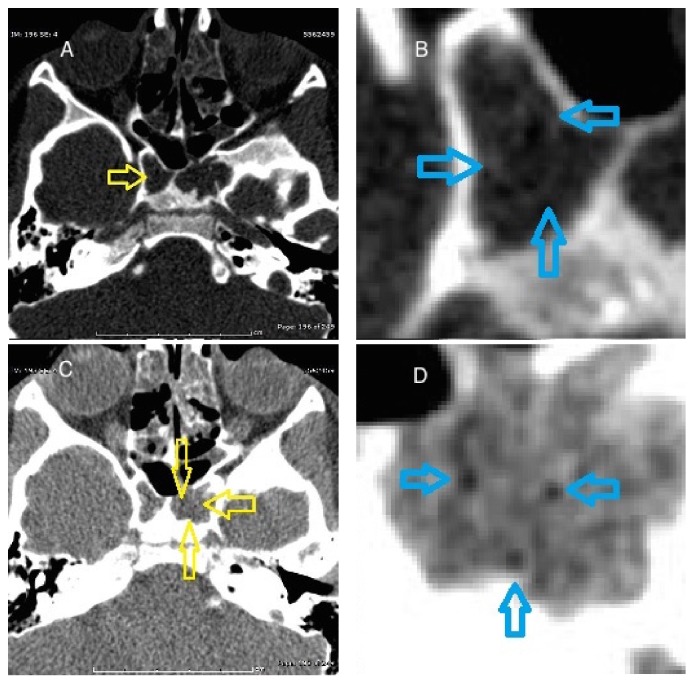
Axial unenhanced CT images through the sphenoid sinus. (A) Curvilinear calcifications (yellow open arrow) are seen in the sphenoid sinus when viewed utilizing bone algorithm. (B) Magnified views demonstrate additional curvilinear calcifications (blue open arrows). (C) When viewed utilizing lung algorithm, there are multiple small foci of fat identified (yellow open arrows). (D) Magnified views more clearly demonstrate these foci (blue open arrows).
